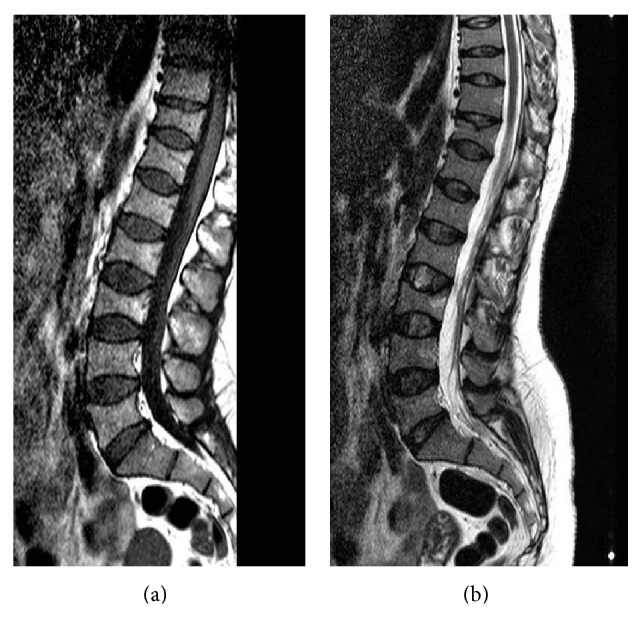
Lumbar MRI de T1 (a) and T2 (b); loss of height in multiple vertebral bodies in various degrees and a biconcave appearance in vertebral bodies.
